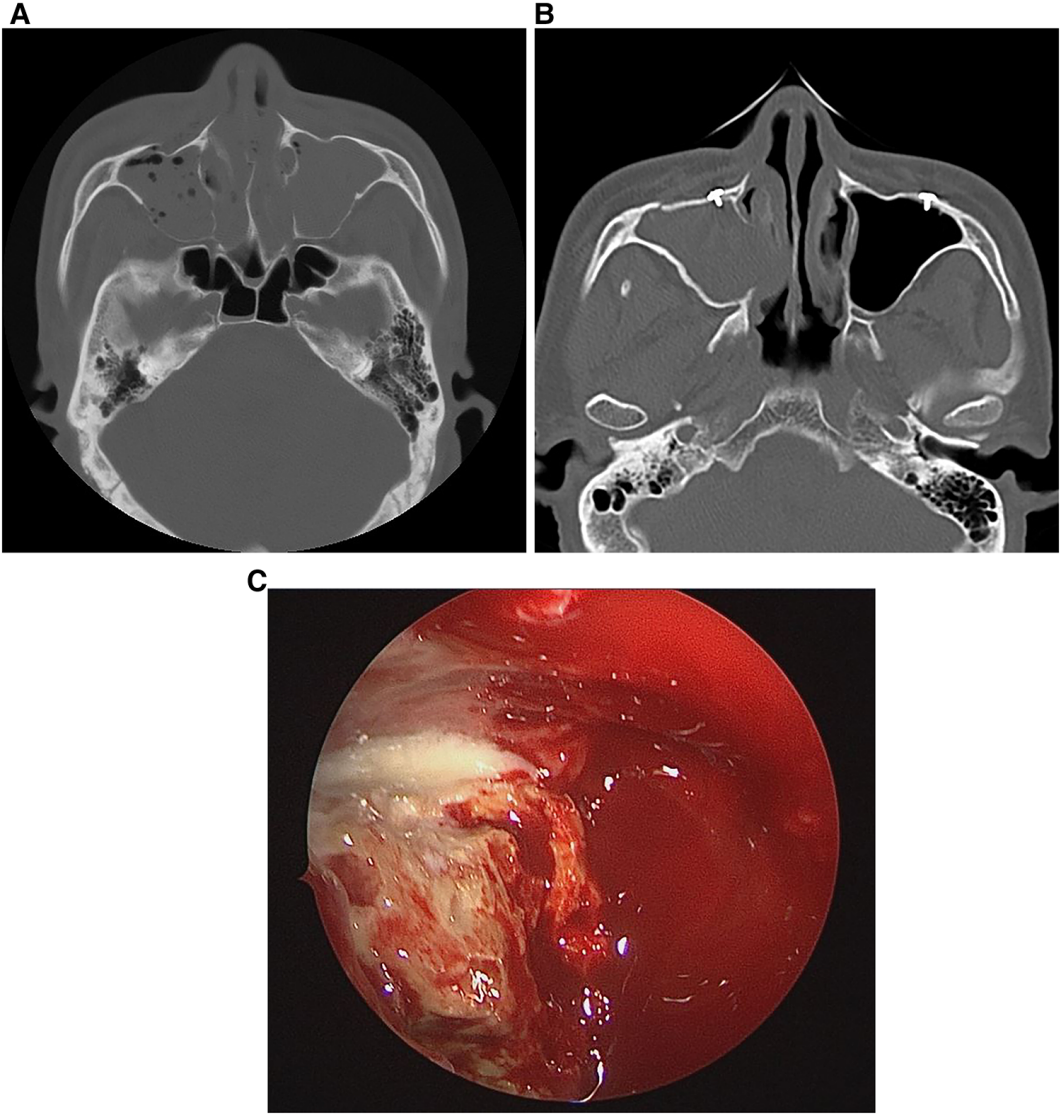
A 54-year-old man with (A) bilateral maxillary sinus fractures due to trauma 18 years prior with fluid and air observed in both maxillary sinuses from bleeding. (B) A month before visiting the hospital, the patient complained of right-sided nasal discharge; CT showed haziness and calcification in the right maxillary sinus, suggesting a fungus ball. (C) Intraoperative photograph showing the maxillary sinus fungus ball.
